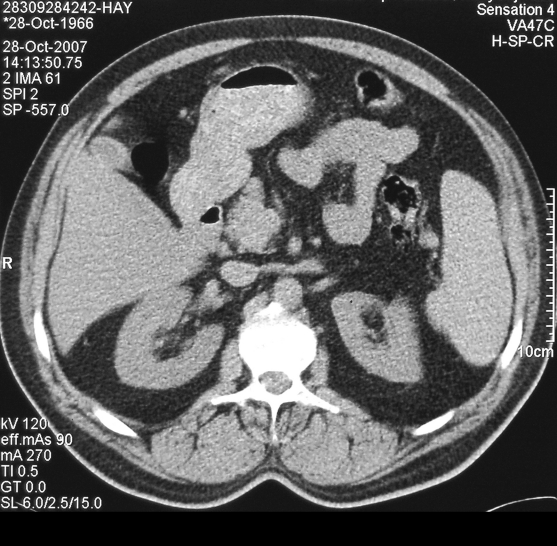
Abdominal CT is completely normal before the bronchial artery embolization.
